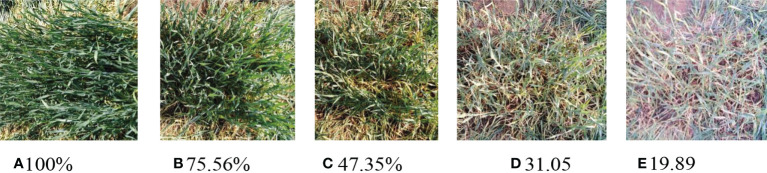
Field ground detection test by ultrasonic sensor. The canopy with different density was formed by randomly and evenly cutting the original wheat four times. (A) 100% (B)75.56% (C) 47.35% (D) 31.05 (E) 19.89.
